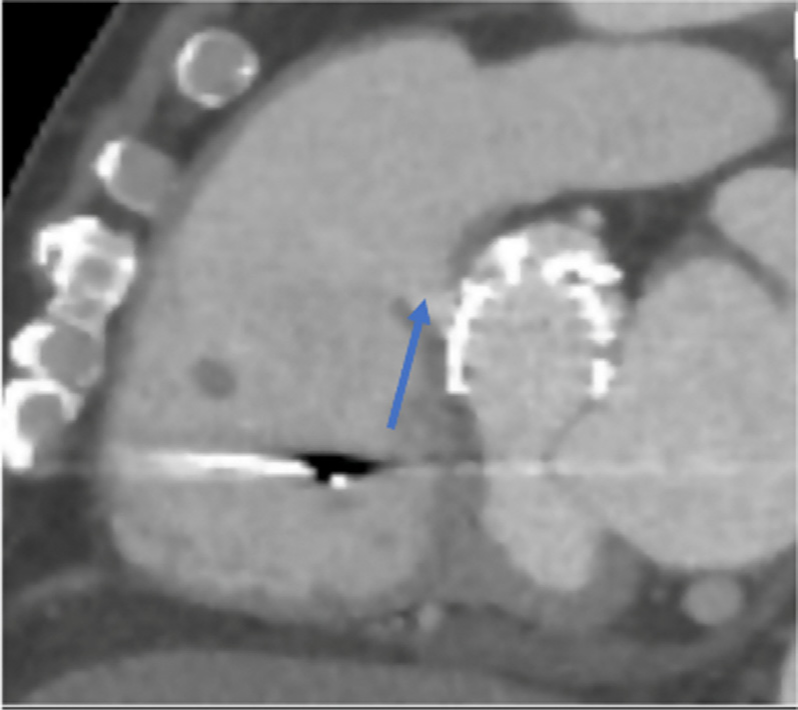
Cardiac Computed Tomography in the Sagittal View The image shows the fistula connecting the aorta near the right sinus to the right ventricle (arrow).
